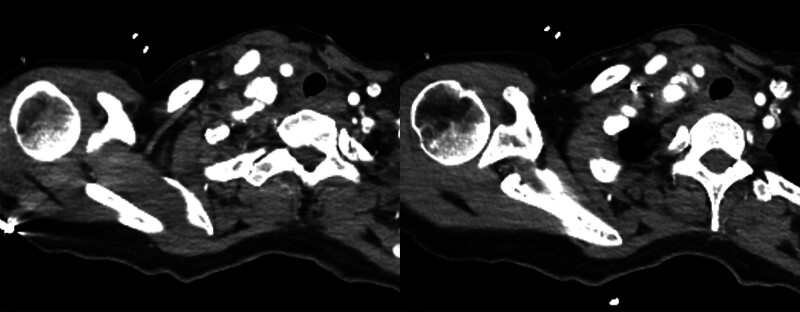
CT angiography showing extravasation of the right subclavian artery. CT = computed tomography.
